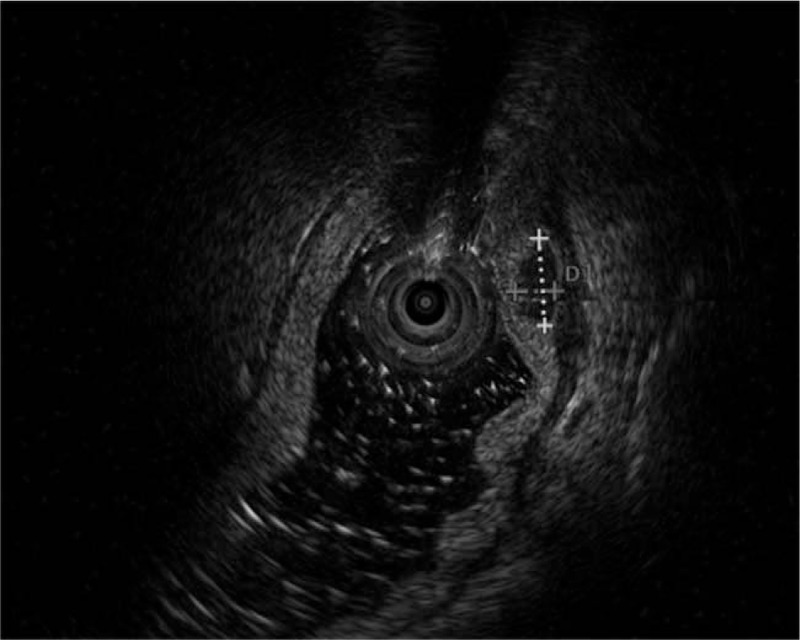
Endoscopic ultrasonography demonstrated that the lesions were almost 0.5-cm homogeneously hypoechoic neoplasms and originating from the submucous layer.
